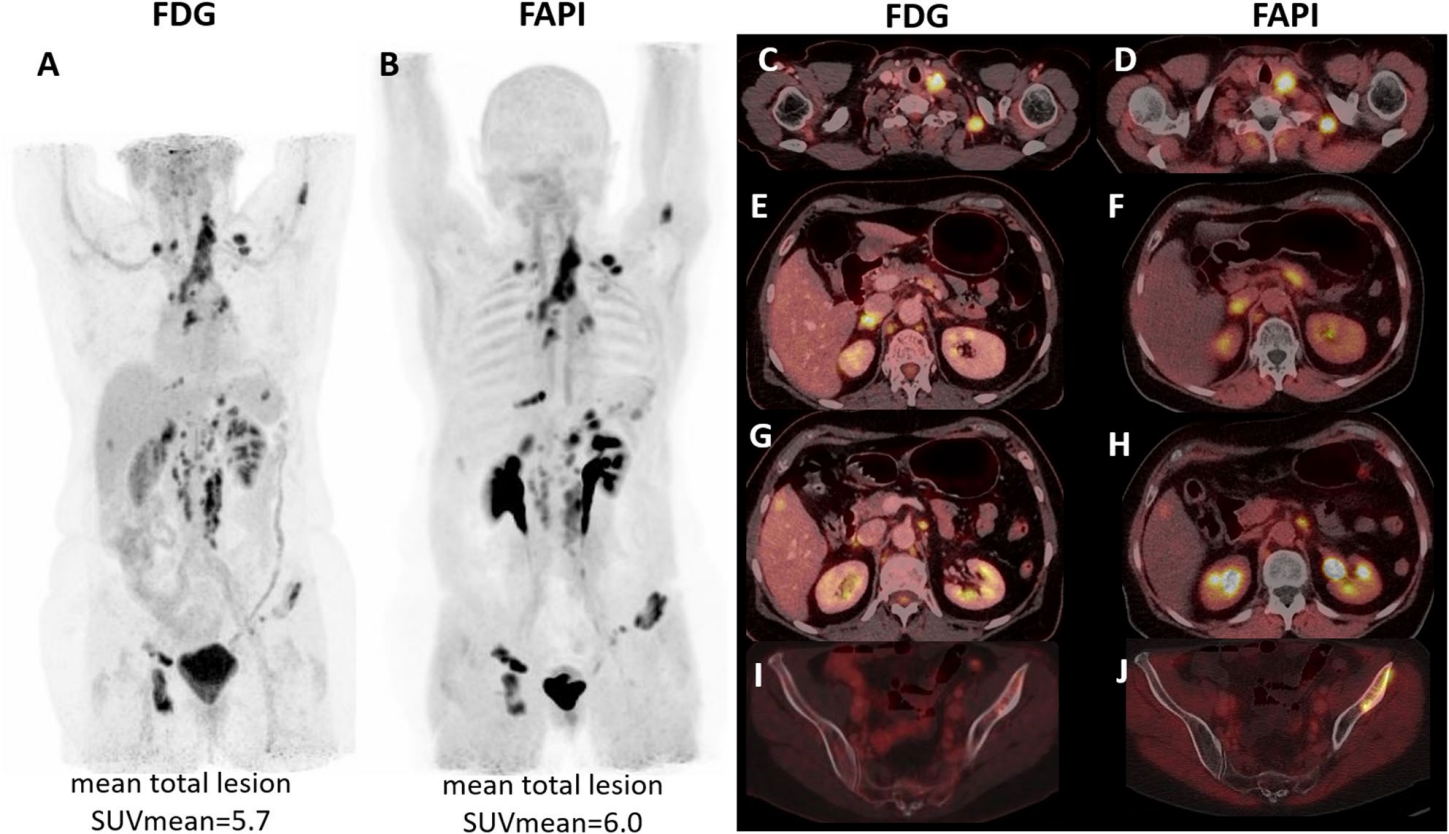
Comparable detection performance of [^18^F]FDG and [^68^Ga]Ga-FAPI-46 PET/CT. 74 years old woman diagnosed with left-sided ductal luminal B-like breast cancer (UICC stage 2a). PET/CT showed pathologic uptake in local lymph nodes (SUV_max_ [^18^F]FDG 11.0 vs. [^68^Ga]Ga-FAPI-46 10.5 - C&D), liver (SUV_max_ [^18^F]FDG 5.3 vs. [^68^Ga]Ga-FAPI-46 3.1), adrenal gland (SUV_max_ [^18^F]FDG 11.4 vs. [^68^Ga]Ga-FAPI-46 8.6- E&F), distant lymph nodes (SUV_max_ [^18^F]FDG 12.7 vs. [^68^Ga]Ga-FAPI-46 14.2 - G&H), bone (SUV_max_ [^18^F]FDG 13.9 vs. [^68^Ga]Ga-FAPI-46 12.2 - I&J), peritoneal (SUV_max_ [^18^F]FDG 8.9 vs. [^68^Ga]Ga-FAPI-46 10.5), and leptomeningeal metastasis (SUV_max_ [^68^Ga]Ga-FAPI-46 4.3, head not included in the [^18^F]FDG PET/CT FOV). Overall lesions presented similar [^18^F]FDG and [^68^Ga]Ga-FAPI-46 uptake (mean total lesion SUV_mean_ on [^18^F]FDG was 5.7 and [^68^Ga]Ga-FAPI-46 was 6.0). A&B - PET maximum intensity projection; C-J - PET/CT fused transaxial plans
